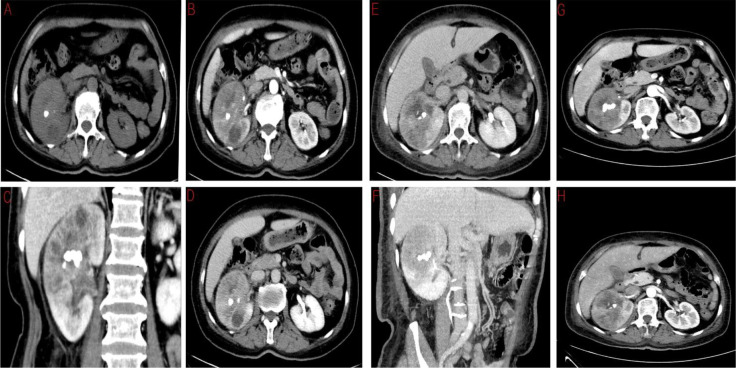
(A) Non-contrast CT scan demonstrating right renal enlargement and nephrolithiasis. (B) Arterial phase contrast-enhanced CT showing heterogeneous enhancement. (C) Venous phase contrast-enhanced CT. (D) Delayed phase contrast-enhanced CT. (E) Bear’s paw sign. (F) The renal veins are slightly irregular. (G) First enhanced CT (Day 4). (H) Second enhanced CT(Day 27).
